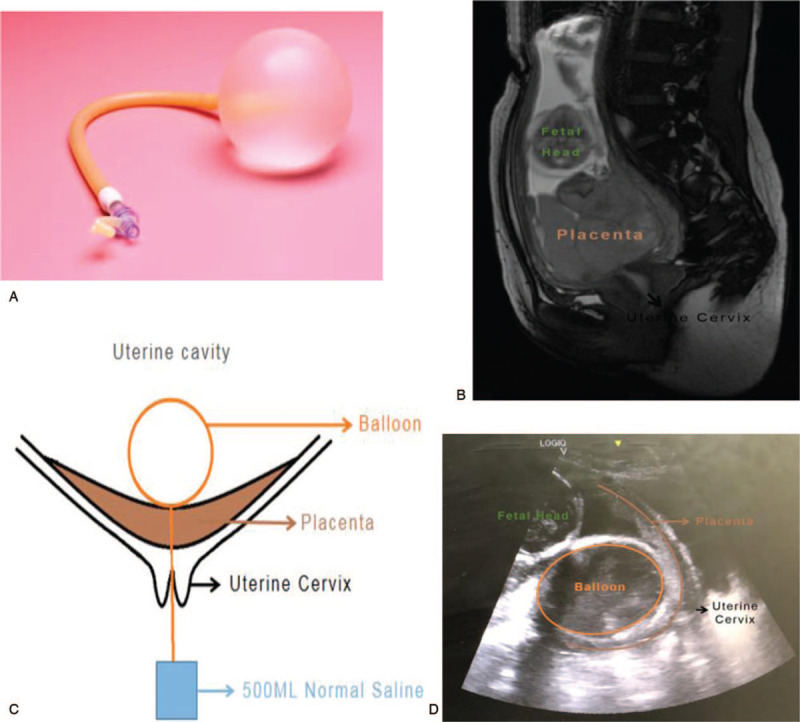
Technical details of using a cervical ripening balloon during pregnancy termination for patients with placenta previa. 1A. A cervical ripening balloon was used. 1B. The exact position of the placenta was evaluated via pre-procedural MRI. 1C. A cervical ripening balloon is inserted through the placenta using toothless oval forceps and was placed in between the fetus and placenta. After the balloon end plug is closed, external traction force (a bag of 500 mL saline) was connected to the balloon to maintain the tension for the cervical ripening balloon to press against the placenta that covers the cervical os. 1D. The positional relationship between the cervical ripening balloon and the placenta is evaluated again by ultrasound. MRI = magnetic resonance imaging.
